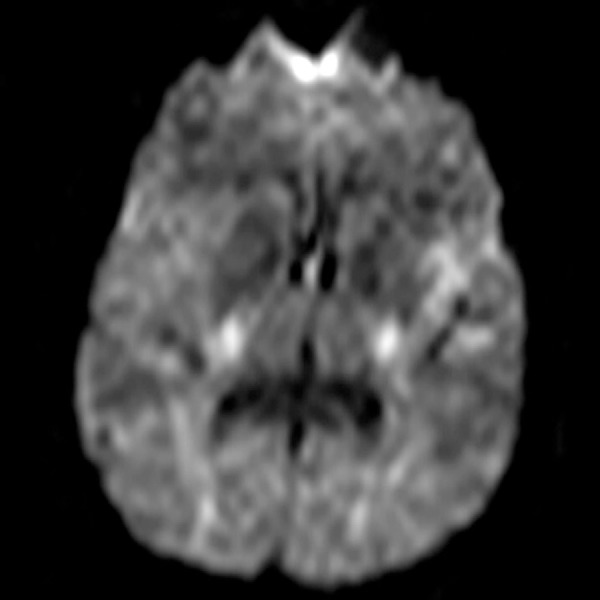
Brain Diffusion Magnetic Resonance Imaging in axial section showing
